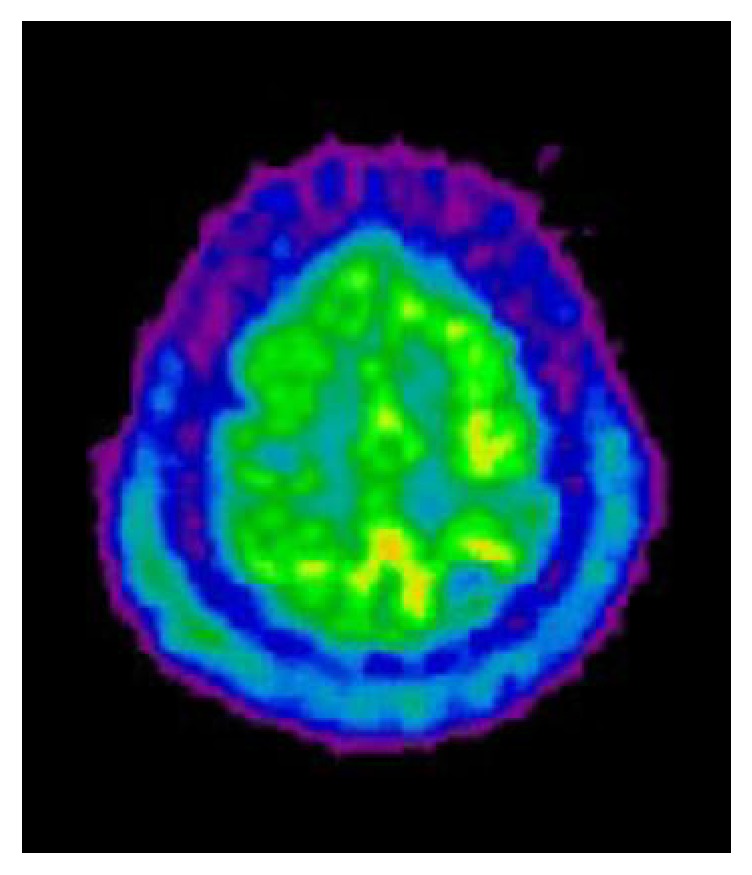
Brain ^18^fluorodeoxyglucose (FDG) positron emission tomography (PET) showing diffuse cortical hypometabolism featured by decreased uptake of the tracer.
